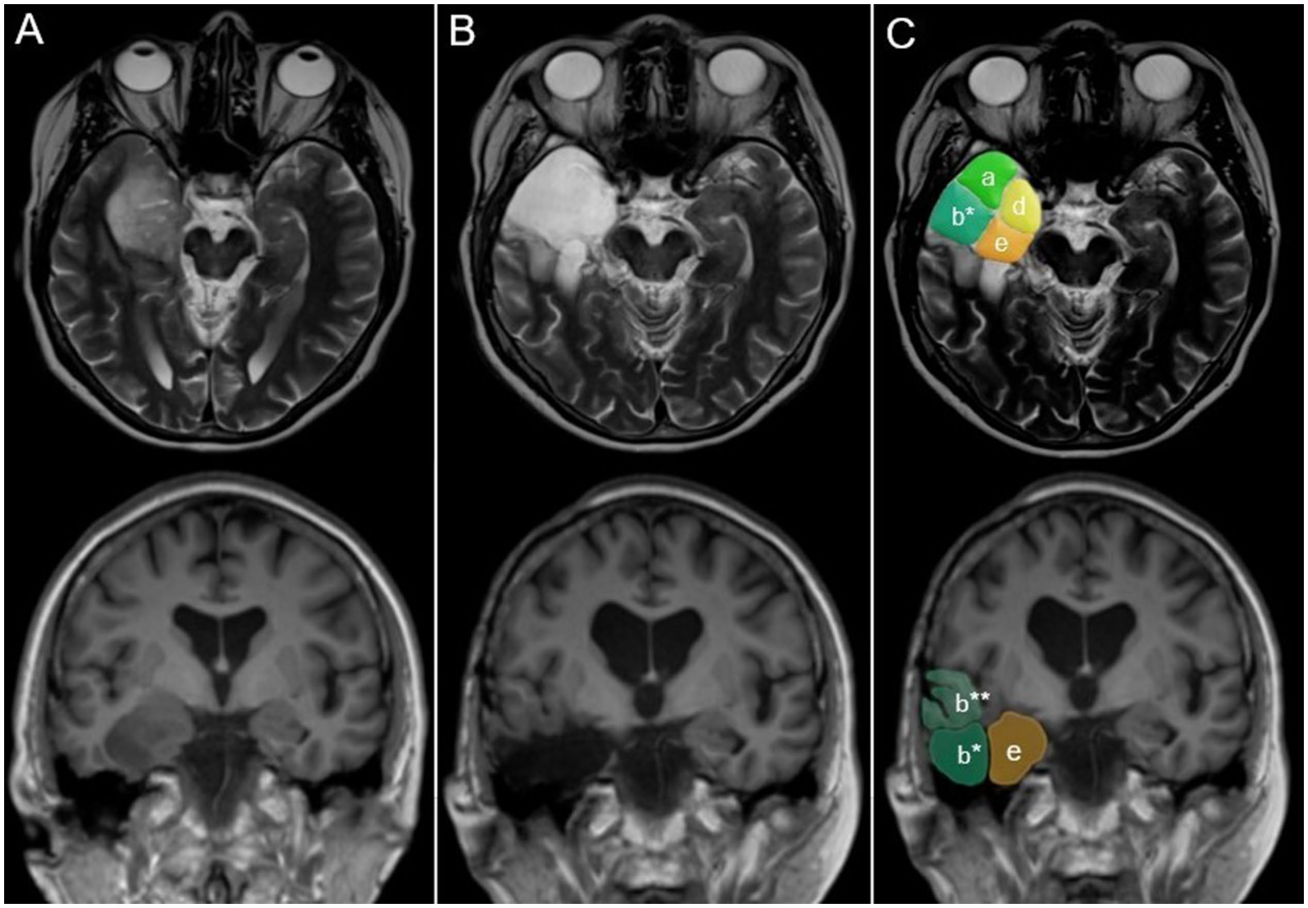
Anatomy-guided surgery for an anaplastic oligodendroglioma CNS WHO grade 3 in a 65-year-old patient. (A) Preoperative; (B,C) postoperative axial and coronal MR images. The tumor primarily involved (d) the anterior and (e) posterior temporo-mesial compartments and (a) the temporal pole. Aiming at both tumor and epilepsy control, the patient had a modified anterior temporal lobectomy, i.e., resection of the inferior part of the anterior temporal lobe (b*; superior part: b) in addition to the compartments obviously infiltrated by the tumor (a,e,d). Compartment designations are the same as in Figure 1.
